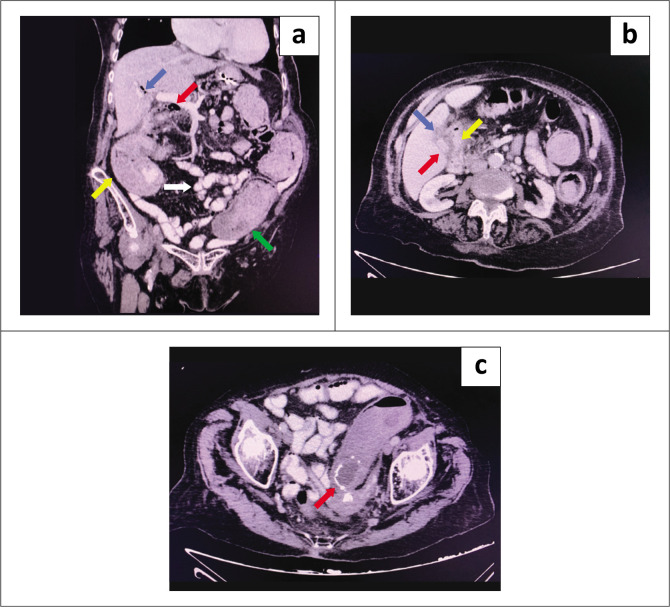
(a) Coronal contrasted CT scan demonstrating intrahepatic duct pneumobilia (blue arrow) and extrahepatic duct pneumobilia (red arrow), dilated ceacum (yellow arrow) and dilated large bowel proximal to sigmoid colon (green arrow) with non-dilated small bowel (white arrow) indicating a closed-loop bowel obstruction. (b) Axial contrasted CT scan demonstrating a shrunken gallbladder (red arrow) with surrounding fat stranding (blue arrow) in the region of the duodenum and hepatic flexure (yellow arrow). No obvious fistula was identified. (c) Axial contrasted CT scan demonstrating ectopic radio-opaque gallstone (oval low-density structure with rim calcification in the lumen of the sigmoid colon [red arrow]) with proximal dilatation of the sigmoid colon.
